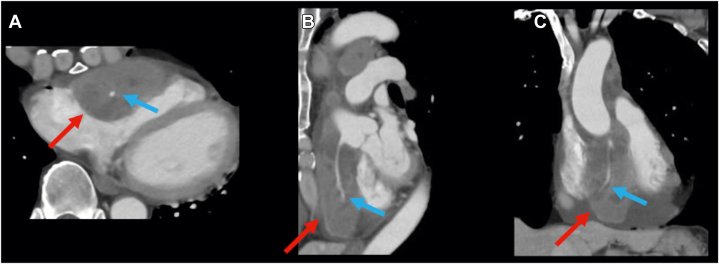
Contrast-Enhanced Computed Tomography Imaging reveals a mass measuring 60 × 35 × 90 mm in the pericardial cavity along the anterior wall of the right atrium and right ventricle, without extension into the cardiac chambers (red arrows) and surrounding the right coronary artery (blue arrows); despite that, blood flow was preserved. (A) Axial view. (B) Sagittal view. (C) Coronal view.
